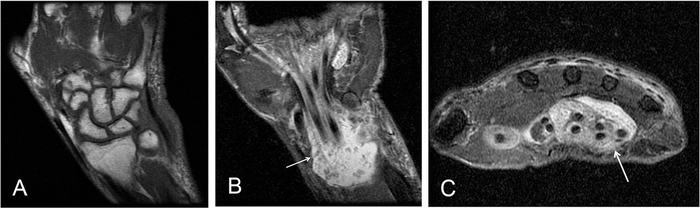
Initial magnetic resonance imaging examination with (A) coronal T1, (B) coronal short T1 inversion recovery (STIR), and (C) axial T2 fat saturation sequences. View A shows no evidence of osseous erosions or marrow-replacing process to suggest osteomyelitis. Views B and C show fluid within the carpal tunnel and associated innumerable low T2 foci, presumed rice bodies (white arrows).
